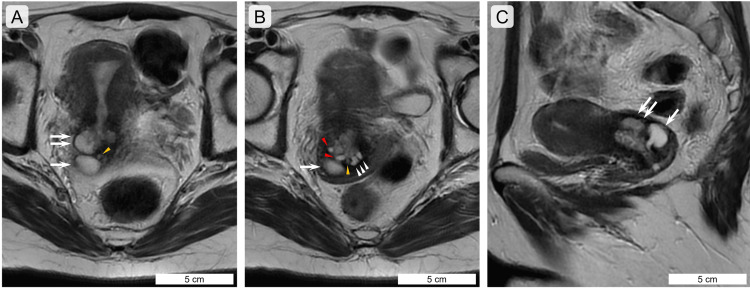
Magnetic resonance imaging photos of multiple Nabothian cysts and tunnel clusters in the uterine cervix. (A)-(C) T2-weighted images (A-B, axial sections; C, sagittal section) of magnetic resonance imaging (MRI) of the uterine cervical lesions. Images (A) and (B) are serial axial sections, and the photograph of (B) shows a more foot-directed section of the photograph in (A). Large, small, high-intensity, and demarcated lesions suggesting cysts are seen in the uterine cervix. In comparison with pathological studies, the two deep Nabothian cysts (NCs) are indicated by arrows and double arrows, and the superficial NCs are indicated by red arrowheads. Most tunnel clusters (TCs) cannot be identified using MRI; however, TCs may be indicated by orange arrowheads as lower-intensity lesions. Two superficial NCs (indicated by red arrowheads) are present on the luminal side of the deep NCs. The scale bars indicate photographs.
